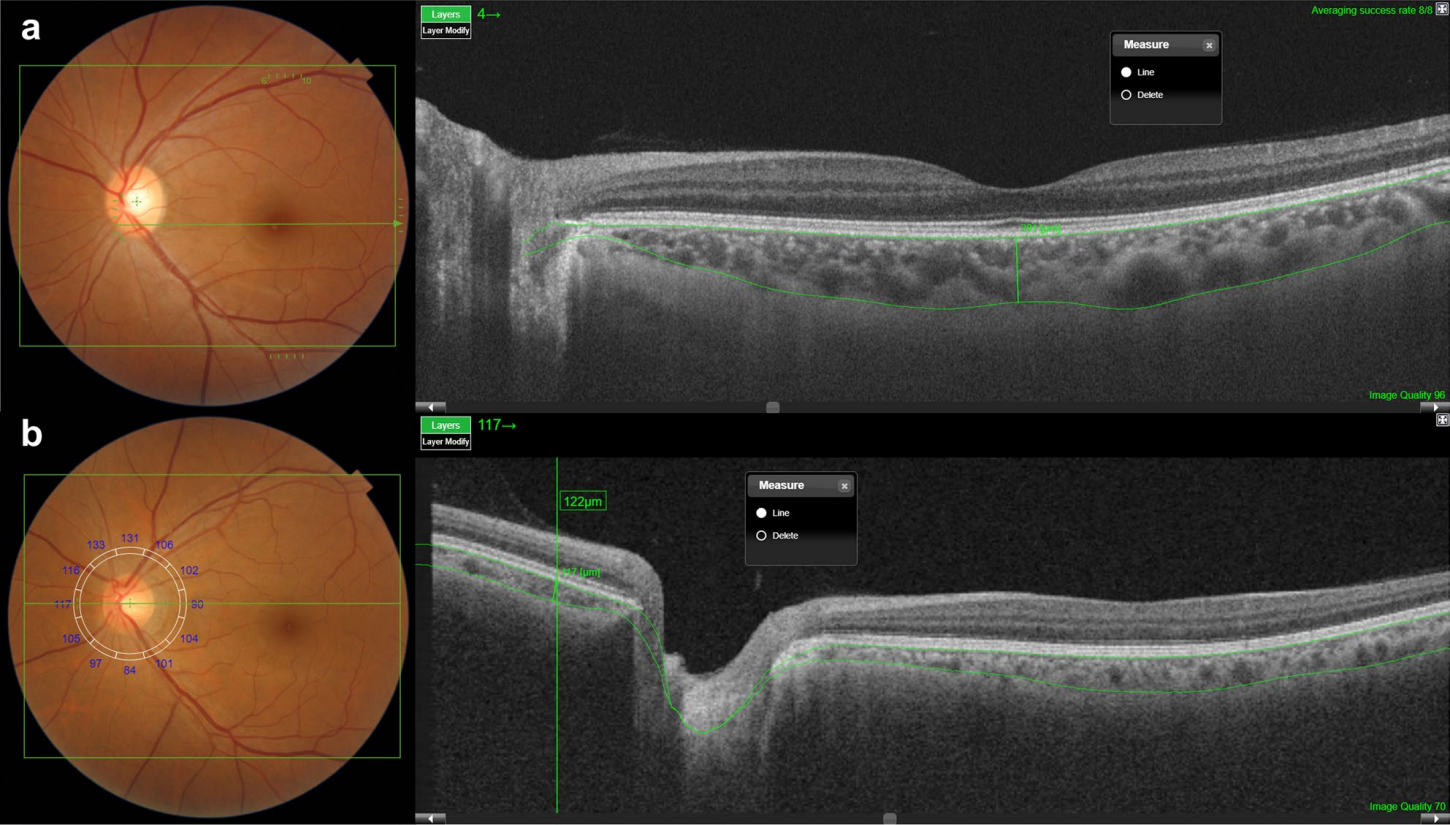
The methods for subfoveal and peripapillary choroidal thickness (CT) measurements. (a) Subfoveal CT was measured from a 9-mm line scan, which was centered on the fovea and averaged 96 B-scans to improve the signal-to-noise ratio. (b) Peripapillary CT was measured from a 12-mm by a 9-mm volume scan image, which was centered between the fovea and the optic disc.
